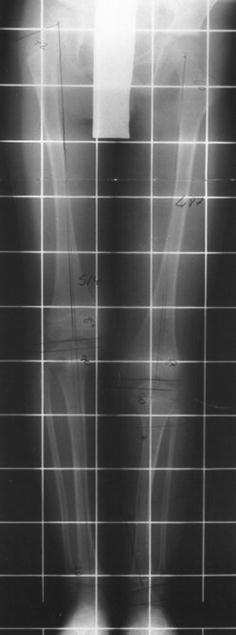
Radiograph of pre-operative mechanical axis
